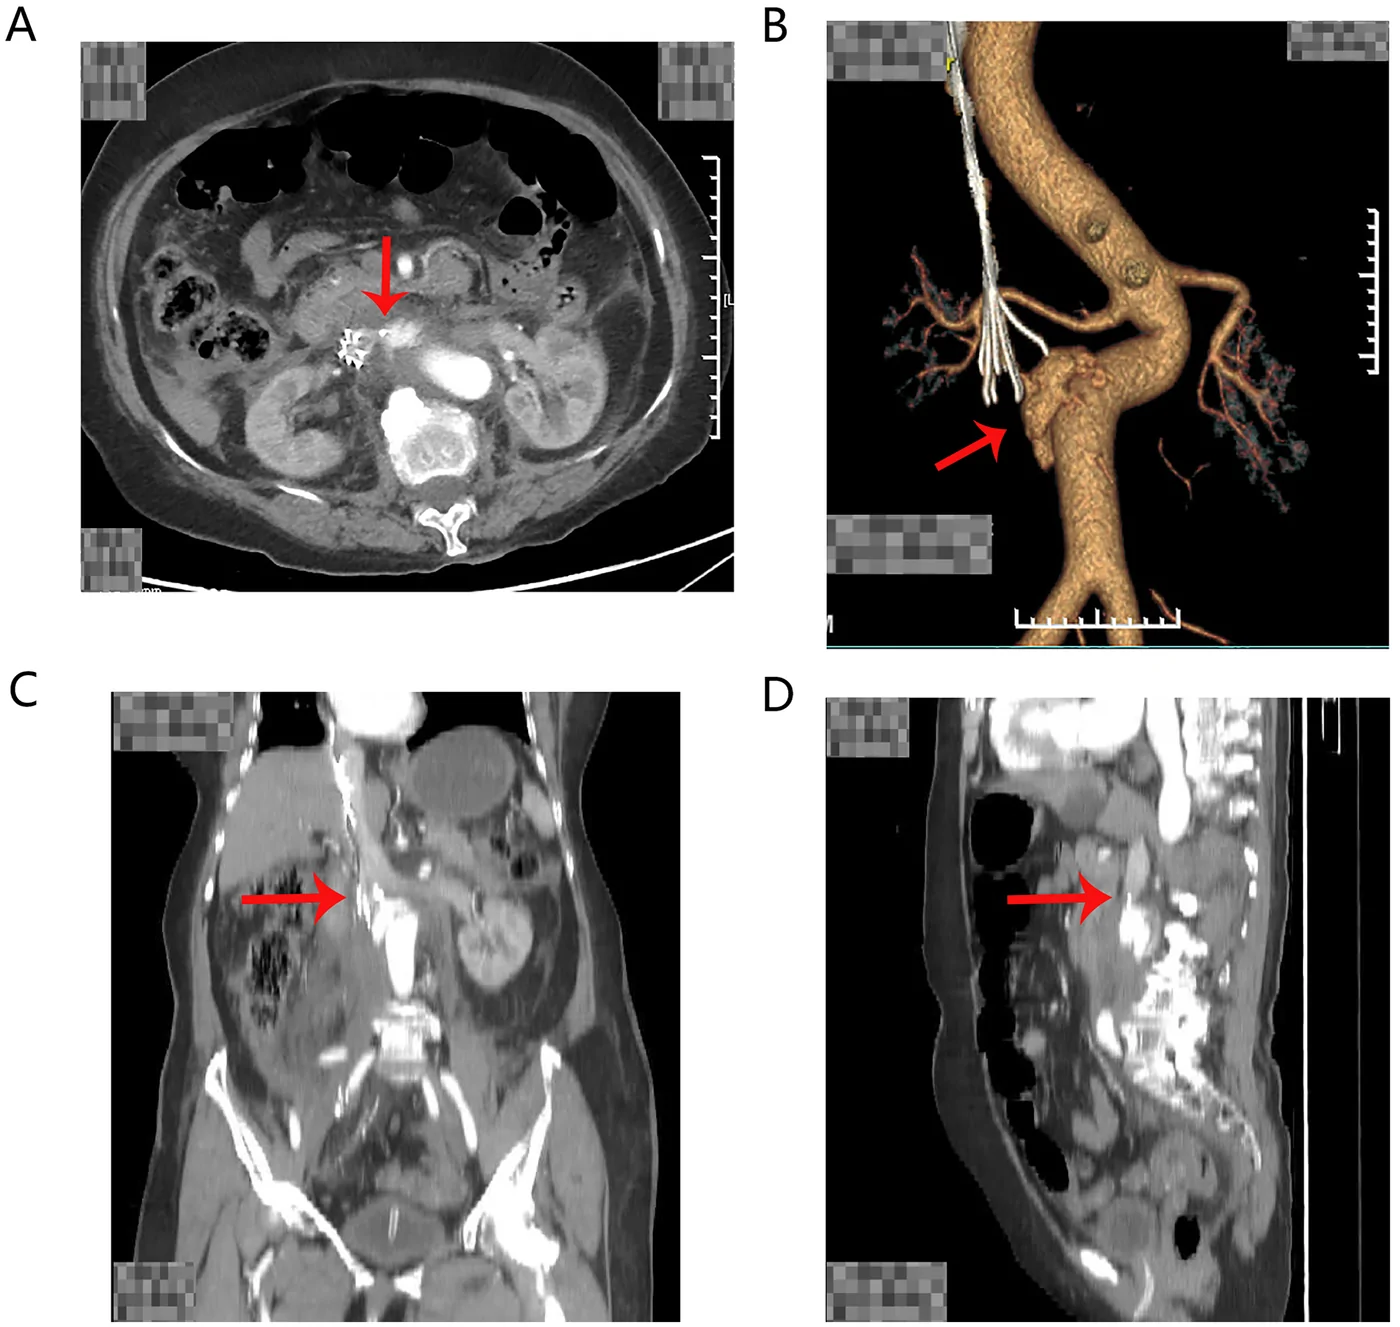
Abdominal aortic pseudoaneurysm: imaging findings. CT images in four planes show that a filter strut penetrated the IVC and entered the adjacent abdominal aorta, resulting in the formation of a pseudoaneurysm (red arrow). (A) Axial image. (B) 3D reconstruction. (C) Coronal image. (D) Sagittal image.
